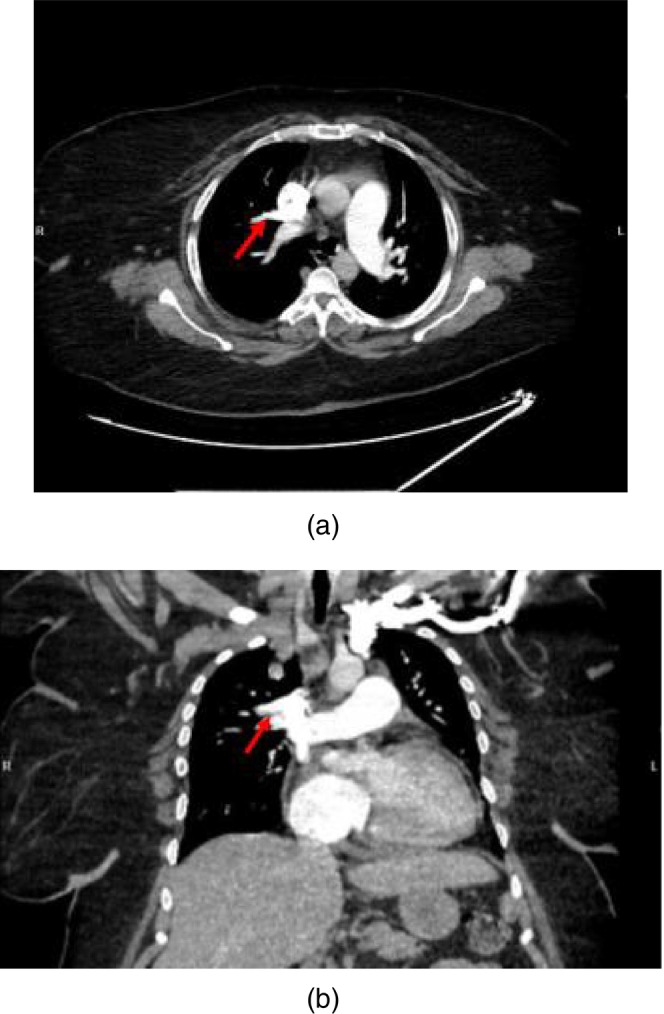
(a) Axial contrast‐enhanced CT chest demonstrating markedly dilated pulmonary artery and right heart chamber consistent with severe pulmonary hypertension. An anomalous vascular channel, seen coursing from the right upper lobe to the superior vena cava, represents partial anomalous pulmonary venous return. (b) Coronal contrast‐enhanced CT chest demonstrating markedly dilated pulmonary artery and enlarged right cardiac chambers consistent with severe pulmonary hypertension.
